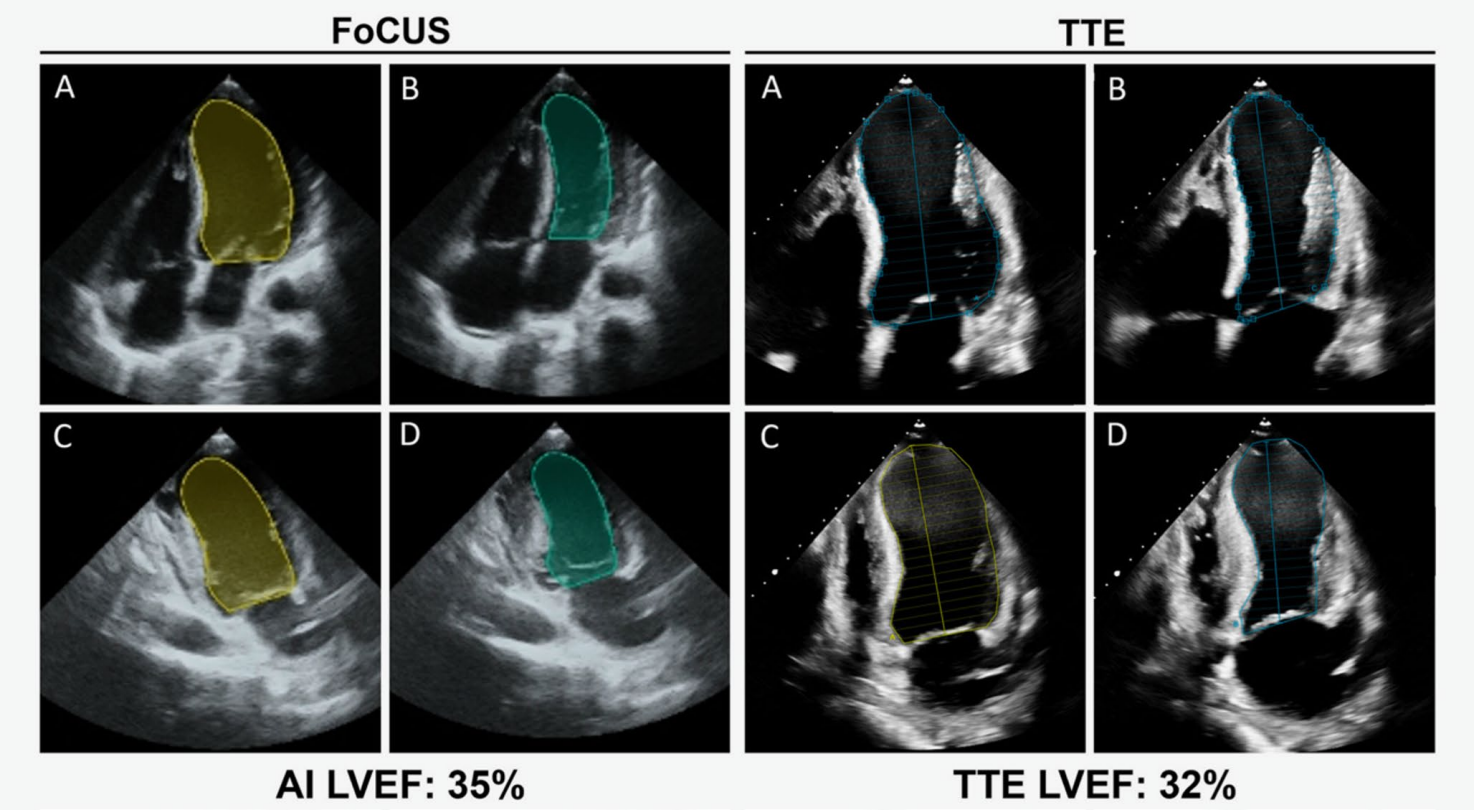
AI-assisted LVEF FoCUS and TTE image acquisition. LV cavity tracings by automated AI and manually by an echocardiographer of the A4C end-diastolic (A) and -systolic (B) frames and A2C end-diastolic (C) and -systolic (D) frames to calculate LVEF. Reproduced from Motazedian et al. [15]
